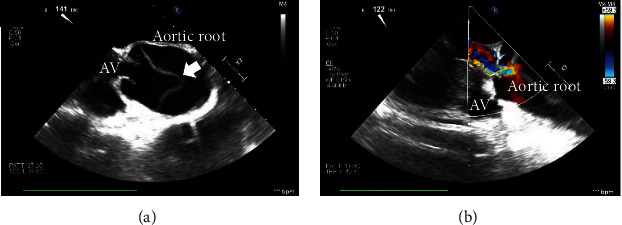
Transesophageal echocardiography shows the intimal flap (white arrow) at the aortic root (a) and aortic regurgitation (b).
